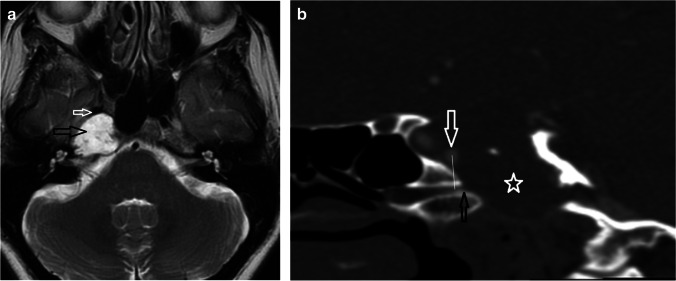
Patient 6. (a) T2w axial image demonstrating the hyperintense right petroclival chondrosarcoma (black open arrow) displacing the carotid artery flow void (white open arrow) anteriorly. (b) Sagittal oblique reformats of the CTA. In the presence of petroclival chondrosarcoma, the pICA (white open arrow) is displaced anteriorly and superiorly from the posterior opening of the VC (black open arrow). The lytic bony destruction of the chondrosarcoma is demonstrated (white star). The craniocaudal measurement is indicated as the vertical perpendicular distance (white vertical line) from the line of the VC to the central point of the pICA cross-section
